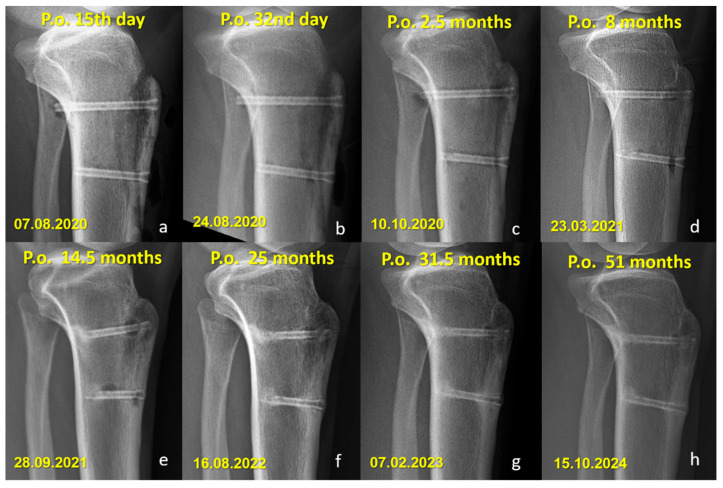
Serial lateral radiographs showing long-term follow-up of a patient who underwent Fulkerson osteotomy fixed with bioabsorbable Mg screws. (a–h) Progressive time points from 15 days to 51 months postoperatively demonstrate bone healing, continued remodeling, and gradual resorption of the magnesium screws.
